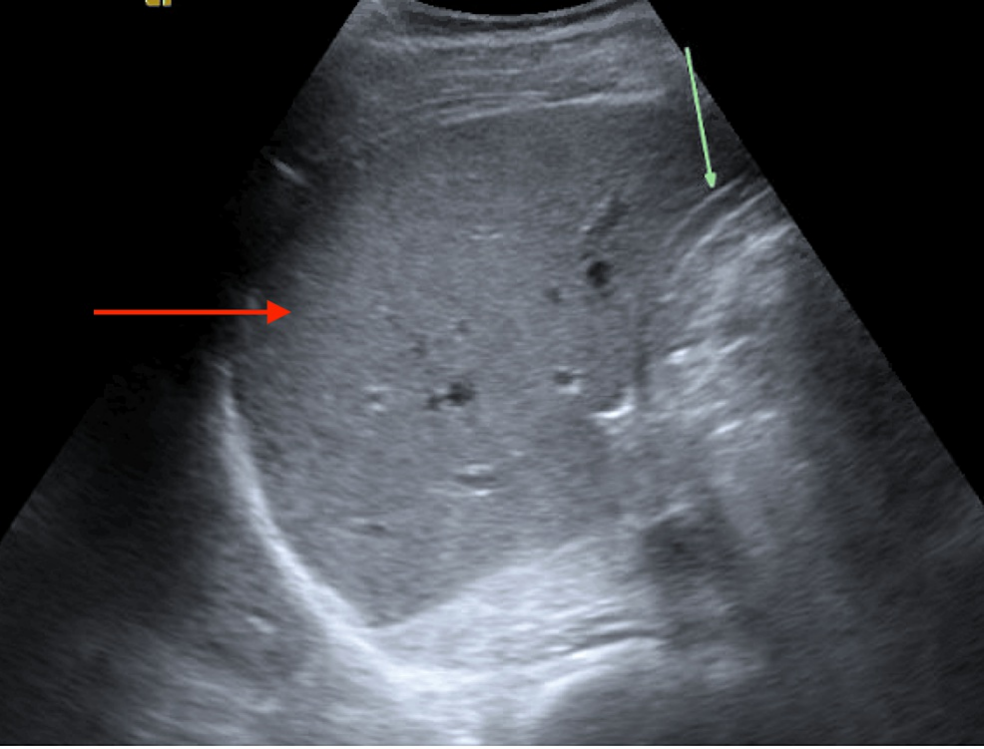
Right upper quadrant ultrasound showing a normal-sized-liver with normal echogenicity (red arrow); the green arrow shows a contracted gallbladder with wall thickening
